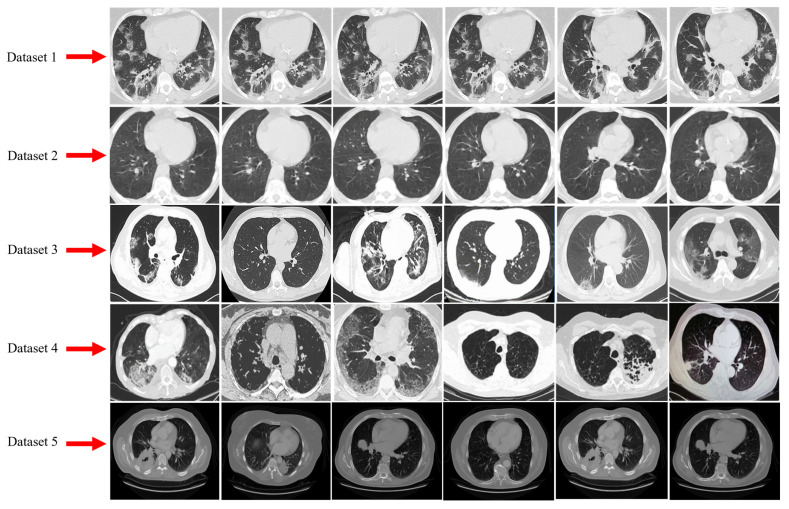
Samples of CT scan images of COVID-19 were collected from Datasets 1 to 5.
